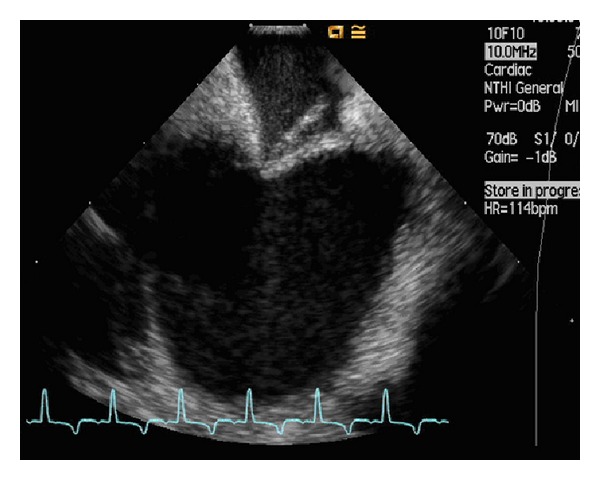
Intracardiac echocardiography (ICE) image of the interatrium septum demonstrating bowing of the midseptum by the Brockenbrough needle. Tenting of the interatrium septum by the Brockenbrough needle is seen, using a 10 MHerz ICE probe. Subject's heart rate of 140 beats per minute without any arrhythmic response to this intervention.
